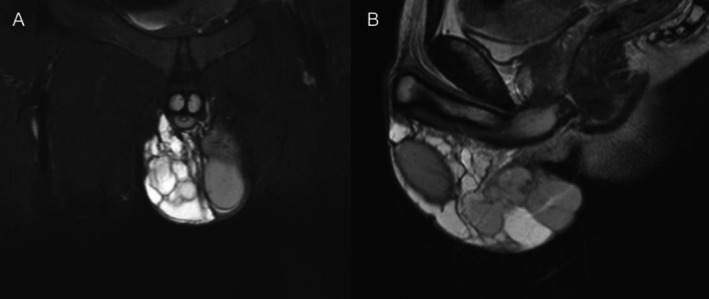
Coronal and sagittal magnetic resonance imaging. The polycystic structure measured 9.3 × 5.7 × 10.9 cm. The right testis and epididymis were compressed, but their shape and size were normal. (A) Coronal plane; (B) Sagittal plane.
